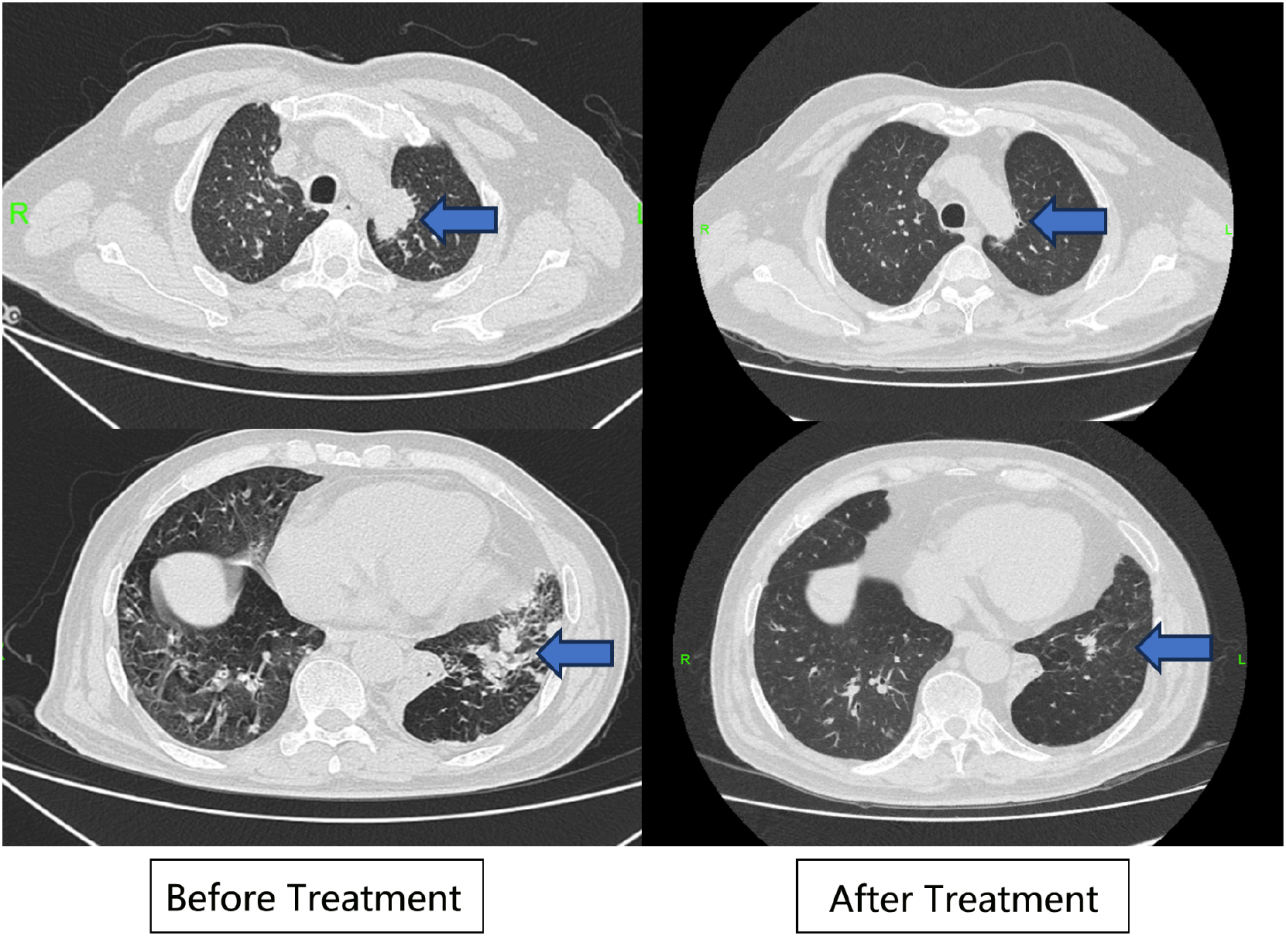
Lung CT. The blue arrows refer to the lesions in lung image before and after treatment.
